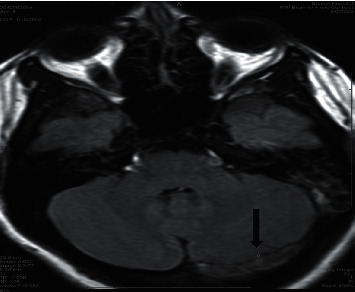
MRI axial flair images show altered SI of the left transverse sinus (arrow).
